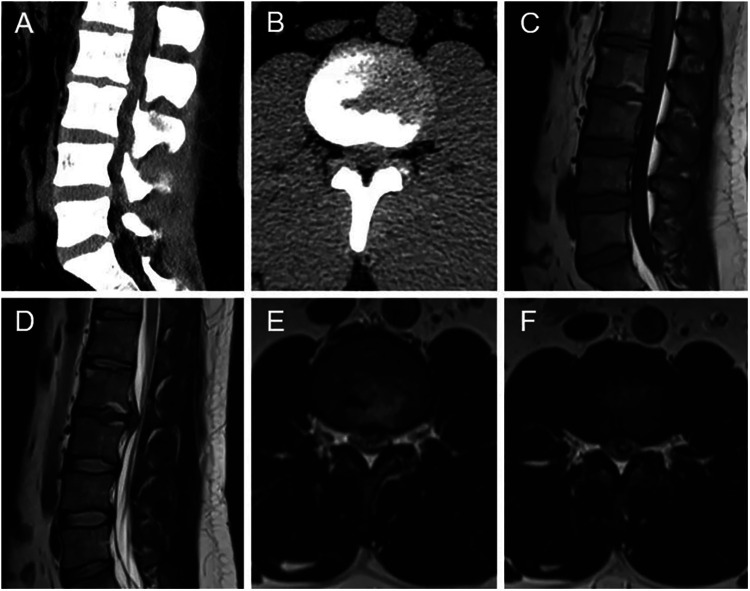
Preoperative radiographic images (A–F). (A,B) CT showed intervertebral disc herniation, loss of disc height, and osteophyte hyperplasia at the L2–L3 level. Sagittal T1-weighted (C) and T2-weighted (D) MRI revealed extruded intervertebral disc, and loss of continuity of the PLL at the L2–L3 level. (E,F) Axial T2-weighted MRI demonstrated the intradural mass.
